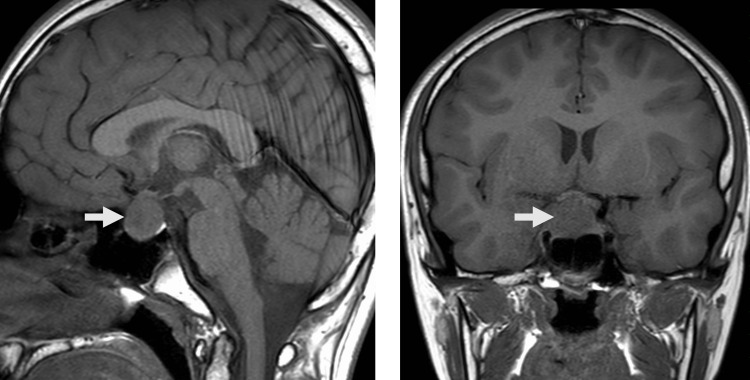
Magnetic resonance imaging (MRI) of the head MRI showing a sellar and supracelluar mass with axial T1-weighted MRI (A) and coronal T1-weighted MRI (B).
